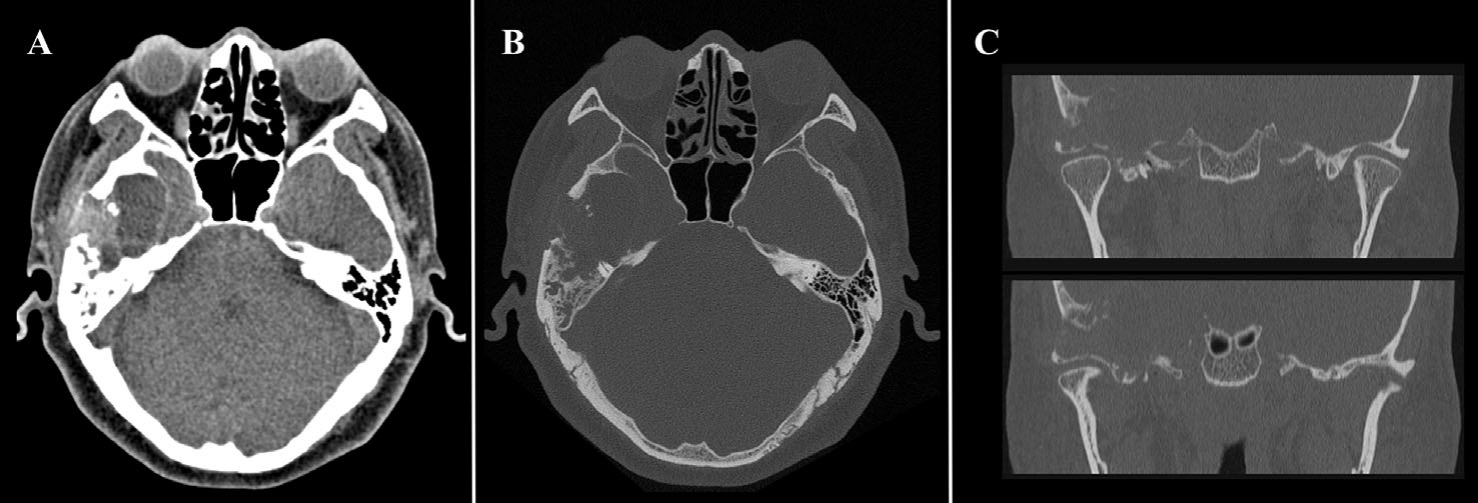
Computed tomography (CT) of a 48-year-old man with diffuse tenosynovial giant cell tumor (D-TSGCT) in right temporomandibular joint (TMJ). (A) A heterogeneous-density mass in the right temporomandibular joint region (soft tissue window, axial view). (B) Involvement of the temporal squamous and petrosal bones (bone window, axial view). (C) Erosion of the temporal bone while the condylar head was preserved (bone window, coronal view).
